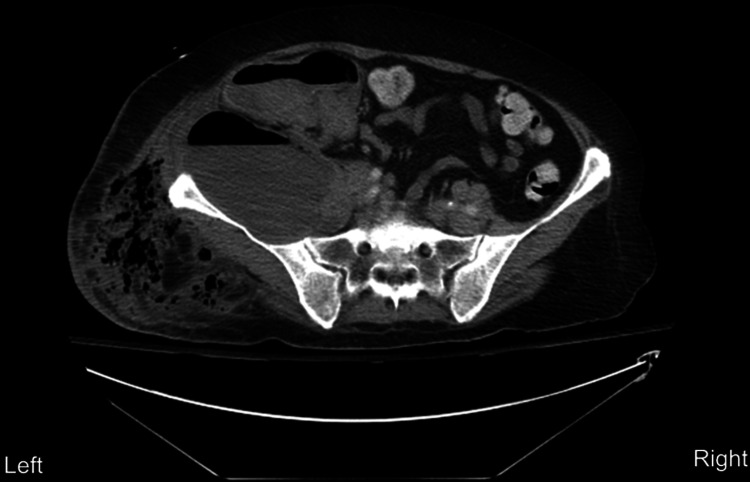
CT scan taken during emergency department admission. The scan was remarkable for a large retroperitoneal abscess with lateral extension through the lower lateral abdominal wall musculature into the subcutaneous tissues. Area of retroperitoneal abscess posterior to the cecum and along the iliac is muscle measures approximately 15 cm in maximal length.
